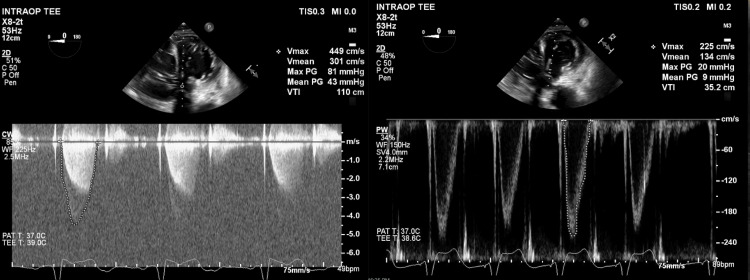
Intraoperative transesophageal echocardiography (TEE) comparing LVOT gradients before (left) and after (right) resection of the AML resulting in improved MG from 43mmHg to 9mmHg Left: Continuous wave doppler across the LVOT before AML resection showing peak and MG of 43mmHg Right: Pulsed wave doppler across the LVOT after AML resection showing reduced MG of 9mmHg LVOT: Left Ventricular Outflow Tract; AML: Anterior mitral leaflet; MG: Mean Gradient
